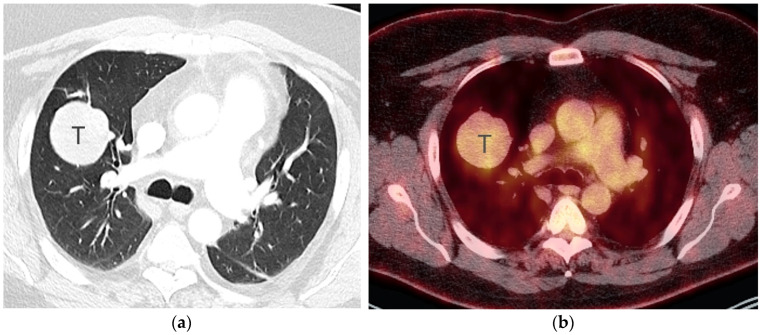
Bronchopulmonary carcinoid. (a) CT shows a 5 cm well-circumscribed solid mass (T) in the right middle lobe. (b) Axial PET/CT shows the right middle lobe lesion (T) has low-grade FDG uptake, similar to that of the mediastinum. Biopsy showed low-grade neuroendocrine tumor.
